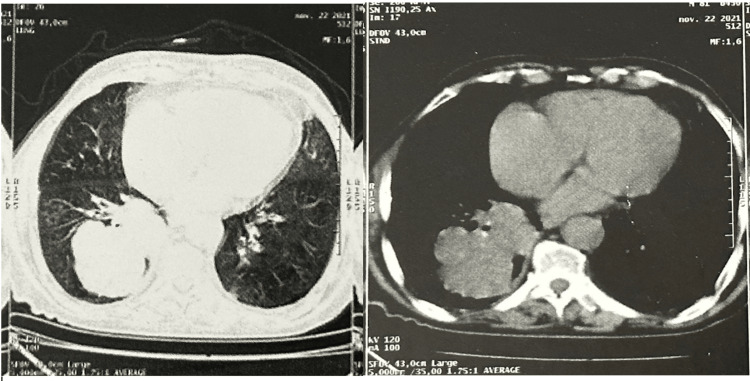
Chest CT scan showing a poorly delimited right post-basal segmental pulmonary lesion, suggestive of a possible malignancy. CT: computed tomography
